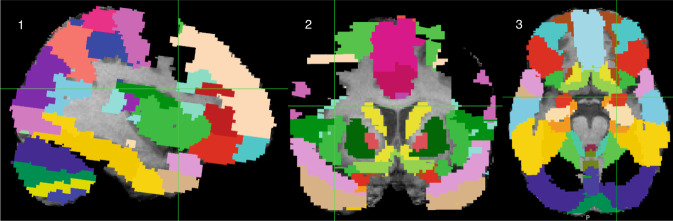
Automated anatomical labeling (AAL) atlas registered to one individual T1-weighted image. Sagittal view of the registered AAL atlas. 2 Coronal view of the registered AAL atlas. 3. Axial view of the registered AAL atlas.
